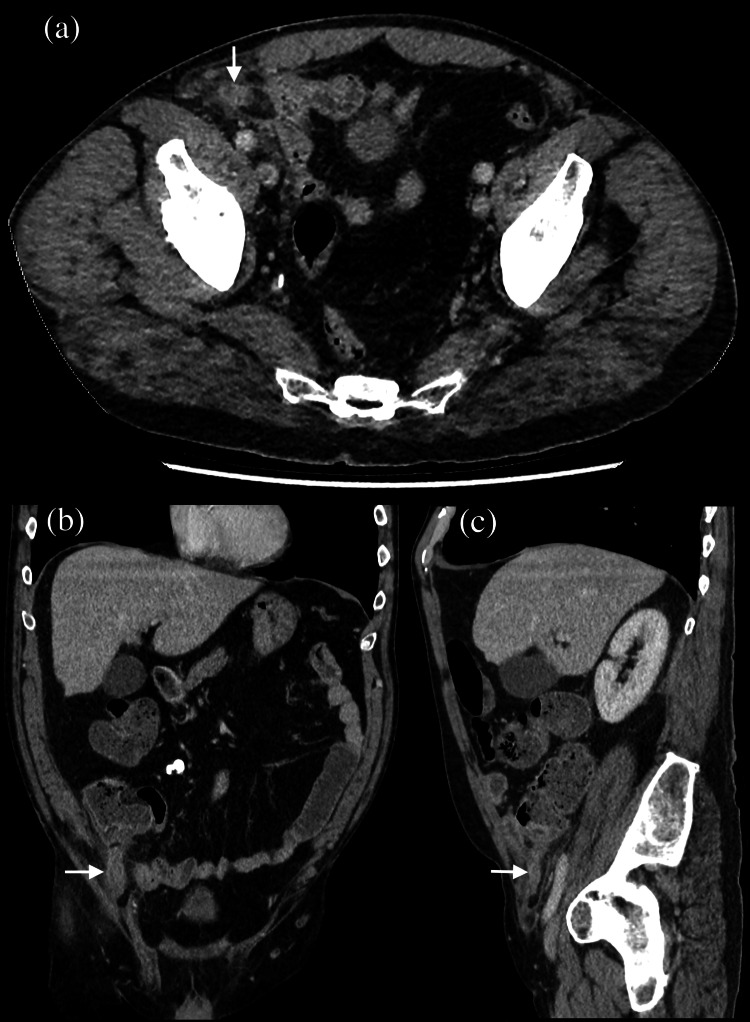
Computerised Tomography (CT) scan of abdomen and pelvis, (a) axial, (b) coronal and (c) sagittal sections in portal venous phase demonstrates terminal ileum, cecum and tip of appendix (white arrows) entering the right inguinal canal and thickened wall of the appendix with periappendiceal fat stranding.
